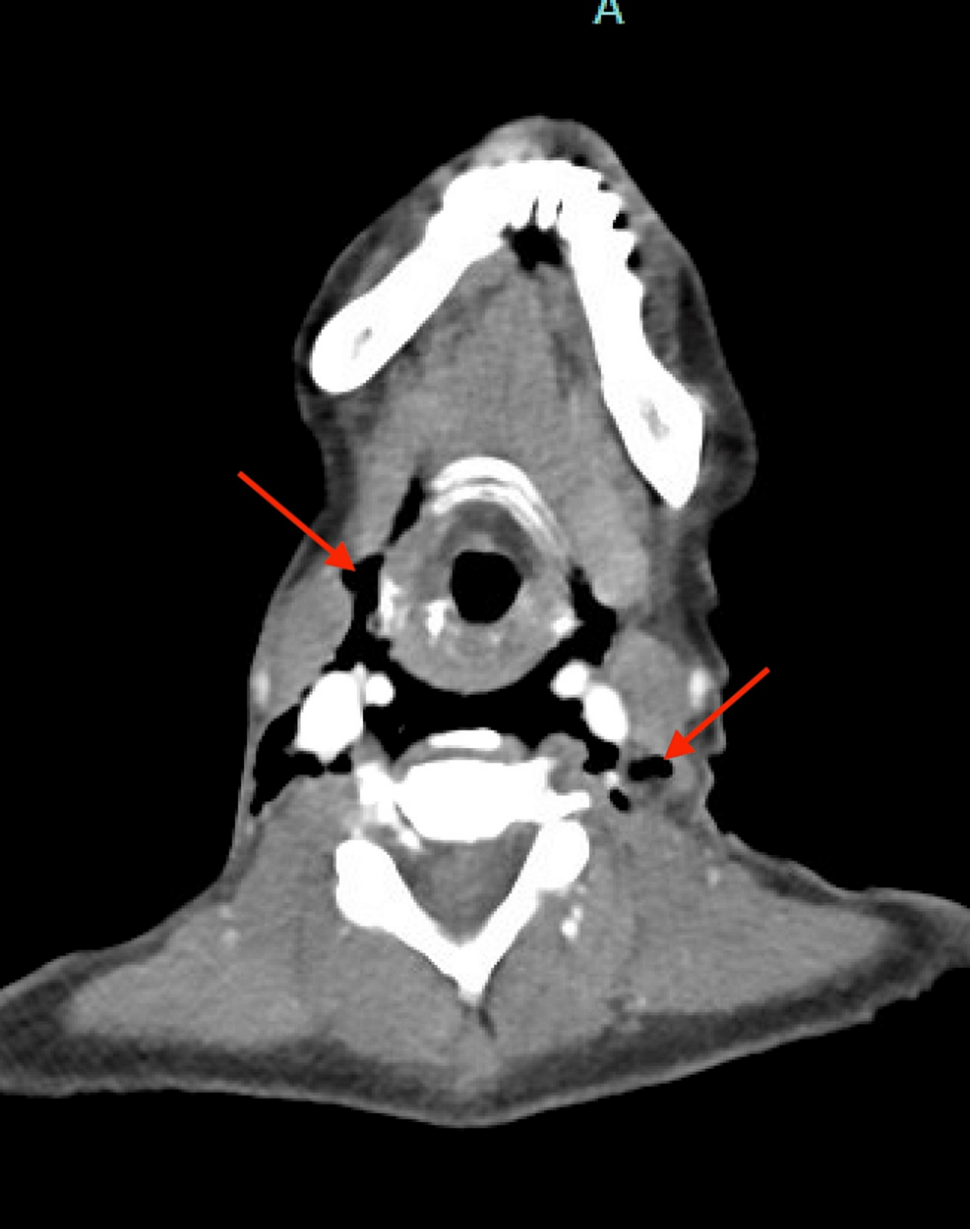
Axial slice of CT neck displaying air in the retropharyngeal space and deep neck spaces (red arrows)
